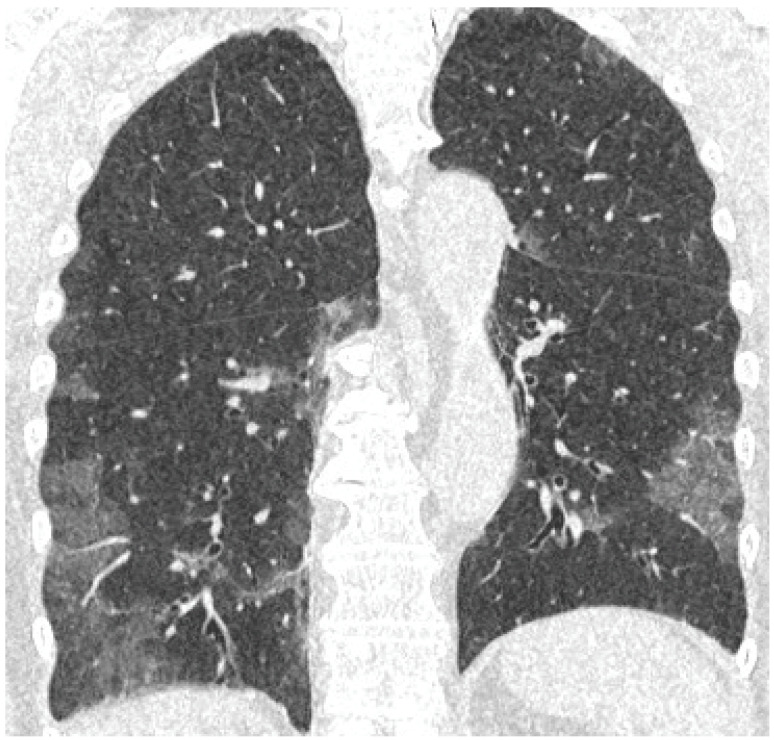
Coronal reconstruction of non-enhanced CT reveals bilateral ground glass opacities in peripheral distribution in lower lobes.
